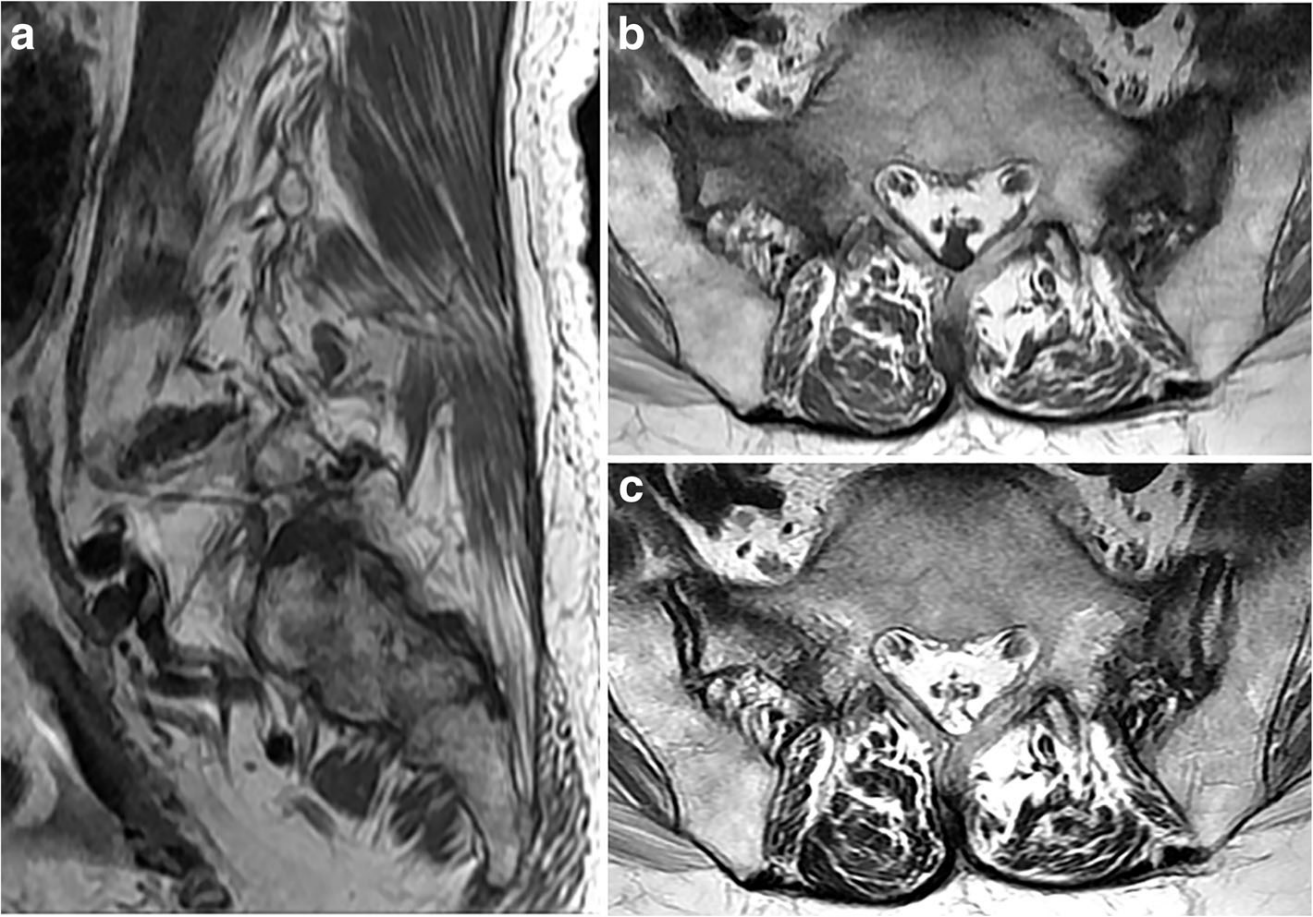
A 68-year-old woman with SIF involving the bilateral sacral alae. a Sagittal T1-weighted MR image shows irregular signal alteration suggesting mild bone marrow edema at the left sacral alae. b Axial T1-weighted MR image at the proximal S1 level shows hypointense fracture lines with surrounding bone marrow edema at both sacral alae. c Axial T2-weighted MR image at the same level shows hypointense fracture line
